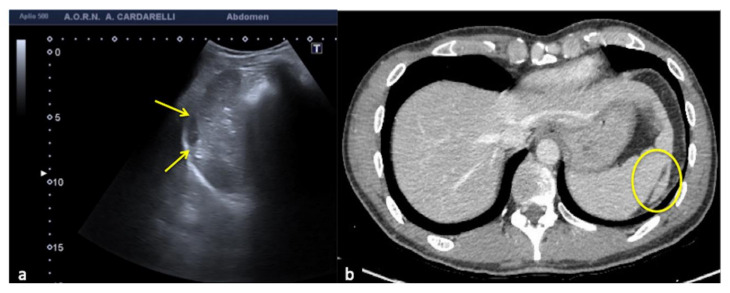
Coronal US scan of the left hypochondrium (a) shows a crescent-shaped hypoechoic area misinterpreted as hematoma (arrows) between the surface of the spleen and the left hemidiaphragm in a 25−year-old man investigated for trauma. On CT scan (b) it appears to be a hypertrophy of the left hepatic lobe with splenic kissing (circle).
